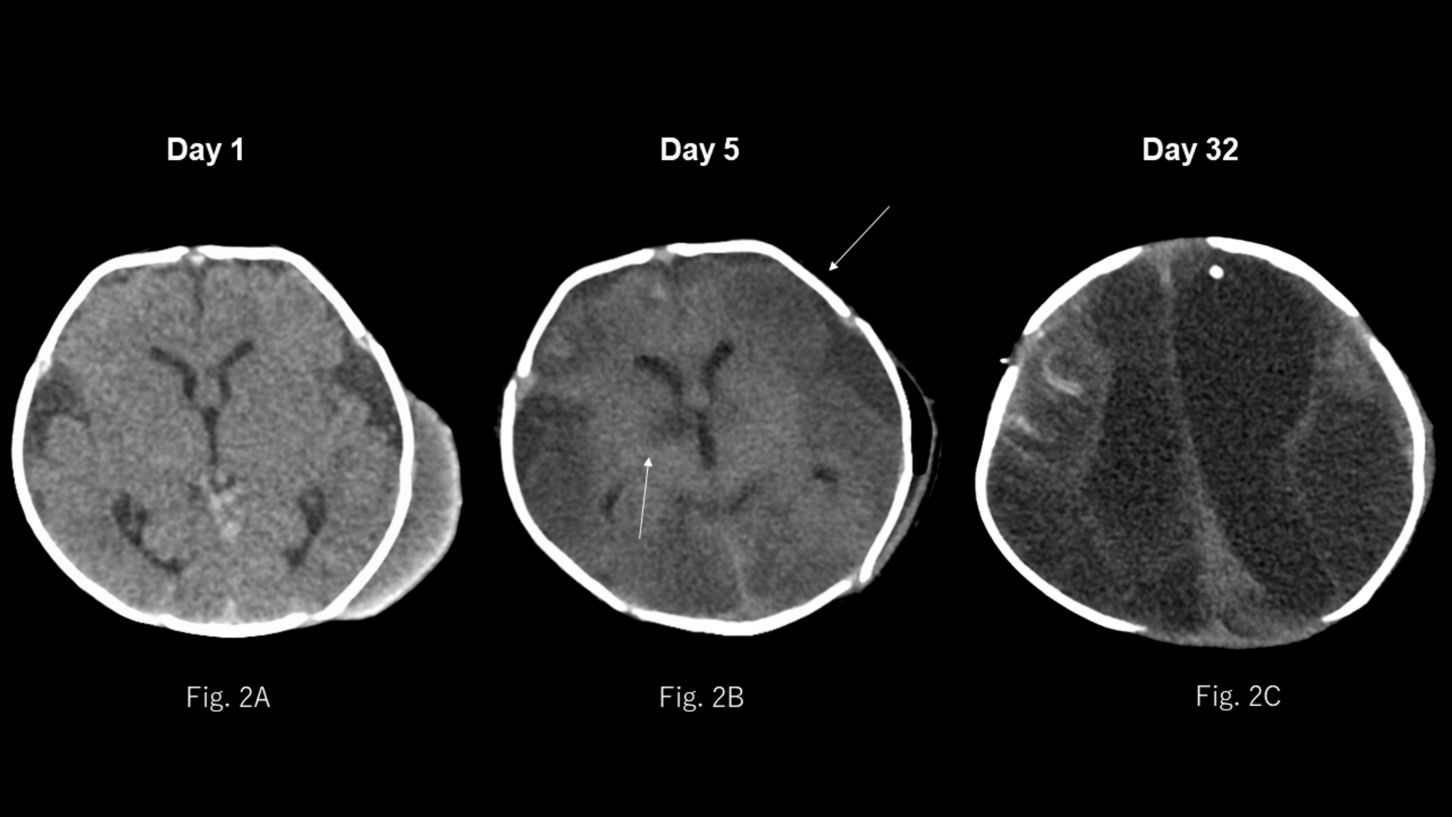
CT images of the head taken without contrast during treatment 2A: On day 1 the cephalohematoma is seen extending from the left temporal to the parietal region with no intracranial abnormalities. 2B: On day 5, the follow-up CT of the head after drainage revealed low-density lesions bilaterally in the cerebral hemispheres and basal ganglia. Subdural fluid collection was observed bilaterally in the frontotemporal regions. 2C: On day 32, laminar necrosis of the right frontal cortex was observed. The CT scan performed on day 32, upon discharge from the intensive care unit, showed bilateral enlargement of the lateral and third ventricles. Extensive low-density areas were observed in both cerebral hemispheres. Cystic encephalomalacia was observed.
